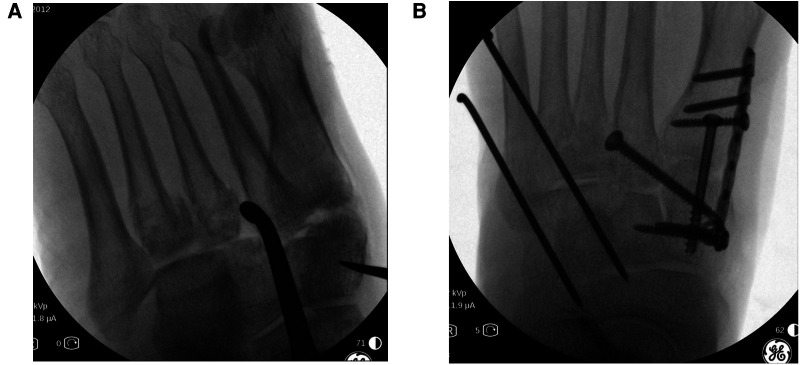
Intraoperative fluoroscopy. The patient's first metatarsophalangeal joint surface is crushed; thus, fusion surgery was performed. The dislocated fourth and fifth metatarsophalangeal joints were difficult to reduce, resulting in significantly increased operative time and intraoperative fluoroscopy. (A) Intraoperative C-arm machine to monitor the metatarsophalangeal joint reduction. (B) Postoperative lateral and anteroposterior radiographs, respectively.
